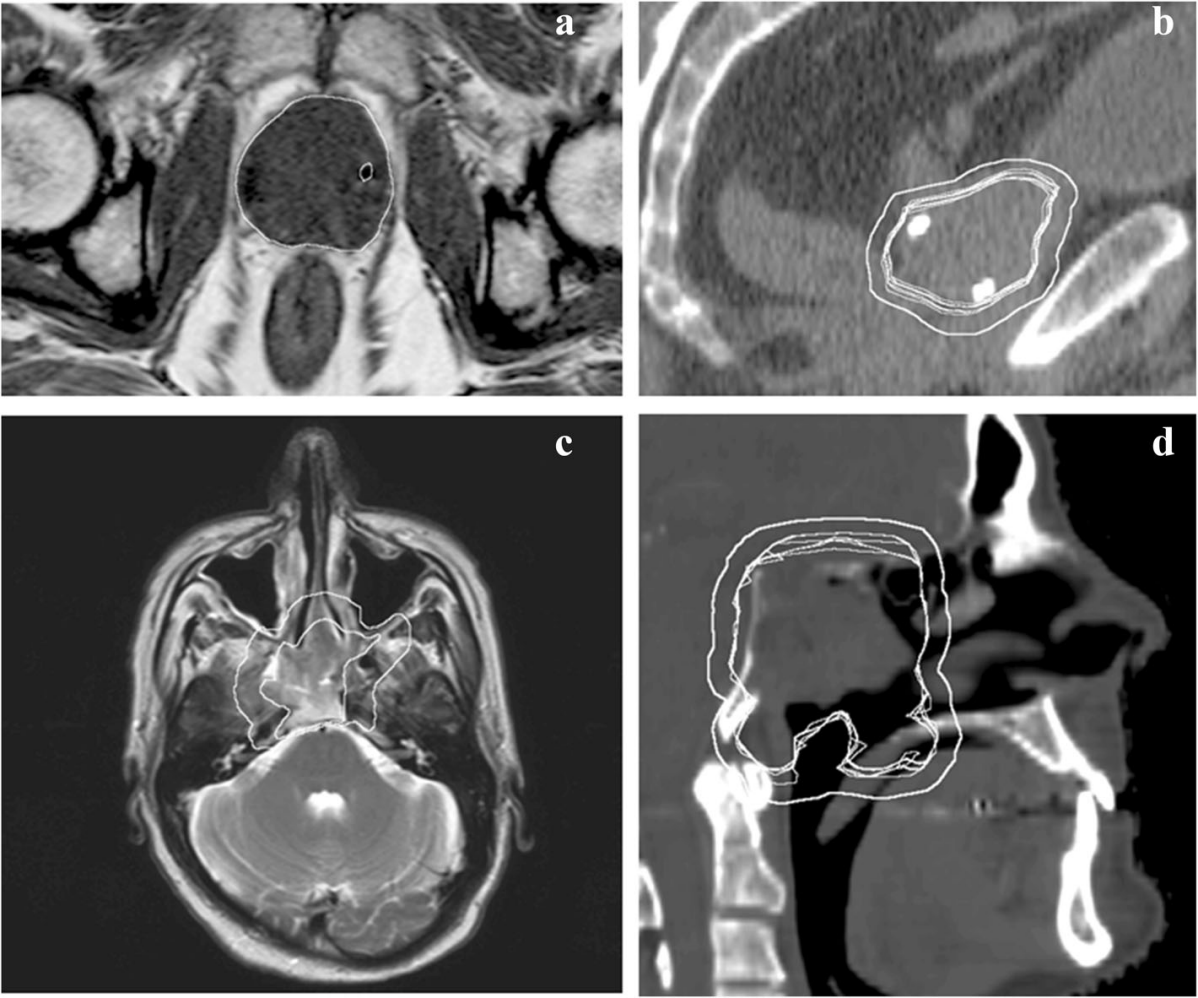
Variability of multiple registrations between MRI and the corresponding CT for prostate (*top*) and nasopharynx (bottom). a: One marker (of three) indicated by a white circle on the axial MRI. b: Two markers shown by the *white dots* on the sagittal CT. The multiple thin *white lines* are the MRI delineated clinical target volume (CTV) transferred to the CT based on the marker registration following department protocol from 7 different observers. The protocol is based on a rigid automatic (mutual information) registration for a limited FOV around the prostate followed by a manual adjustment to match the markers in the three planes. The outermost white line was the planned target volume (PTV) applied. The data are taken from reference [59]. c: The gross target volume (*inner*) and CTV (*outer*) as delineated on the axial MRI. d: The multiple thin *white lines* are the MRI based CTV transferred to the CT by 6 different observers (sagital CT slice shown). The registration is based on a rigid automatic (mutual information) registration followed by a manual fine adjustment. The outermost *white line* was again the applied PTV. The data are taken from reference [60]
